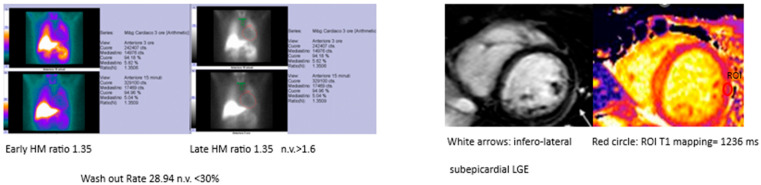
Example MIBG and MRI findings in the same patient.
